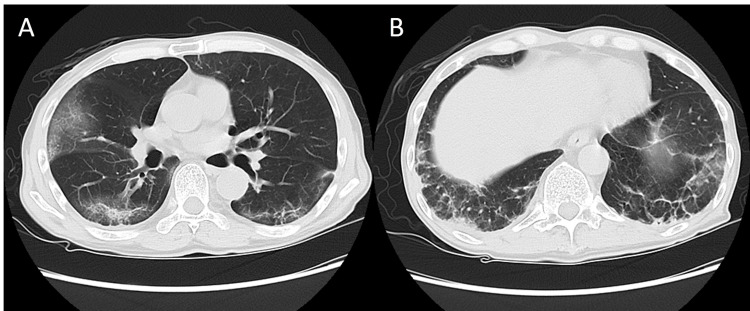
Chest CT in case 2 Chest CT showing GGO in the right middle lobe (A) and a linear shadow and GGO in the lateral and dorsal lower lobe (B).
